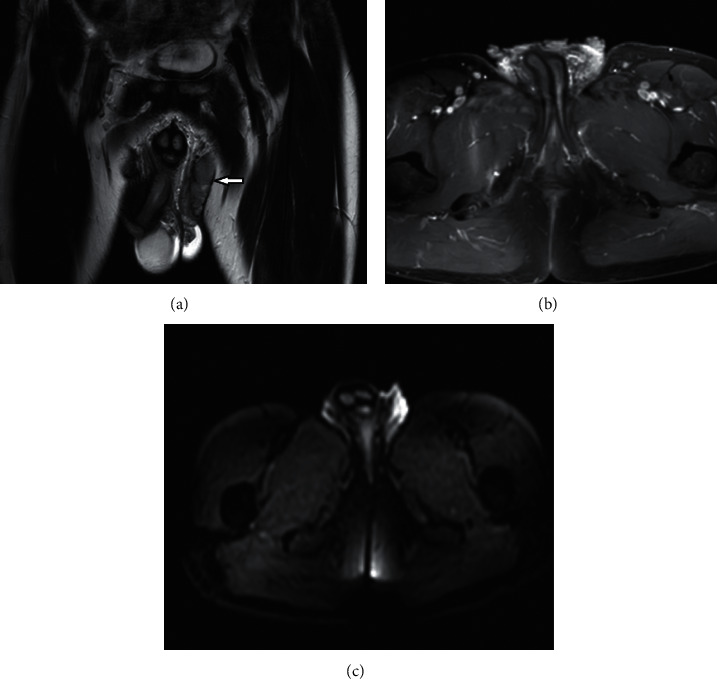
Inguinoscrotal MRI showing the exo- and endophytic inguinoscrotal tumor with intermediate signal intensity on T2-weighted sequences ((a), arrow) enhanced ((b), star) with diffusion restriction (c).
